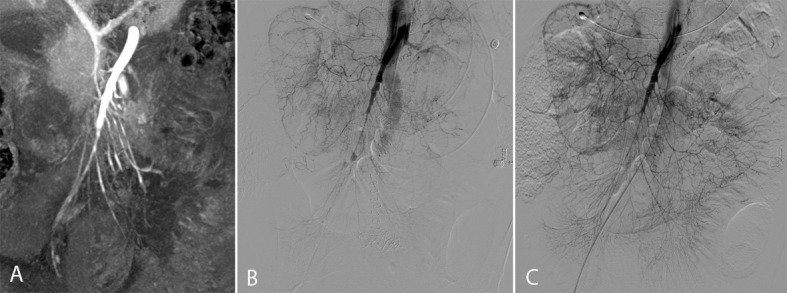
Non-occlusive mesenteric ischemia before and after endovascular vasospasm therapy. Reduced arterial diameter and caliber irregularities in the middle segment and branches of the SMA in CT angiography due to vasospasms from non-occlusive mesenteric ischemia (A). DSA (B) confirms the CT finding. Note the retrograde flow of the contrast agent into the aorta caused by increased vascular resistance in the SMA. Caliber irregularities are reduced after endovascular vasospasm therapy with i.a. prostavasin (C).
